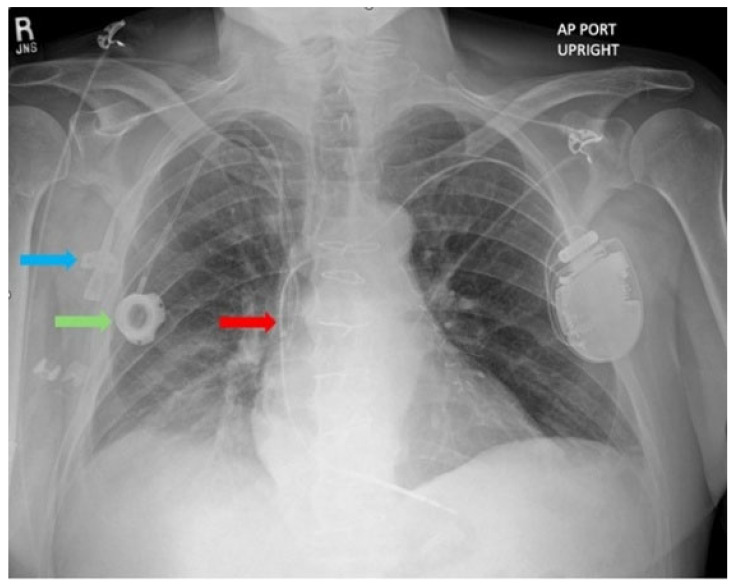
Chest X-Ray. Blue arrow: Peripherally inserted central catheter; Green arrow: Central venous catheter; Red arrow: Single chamber intracardiac defibrillator lead.
